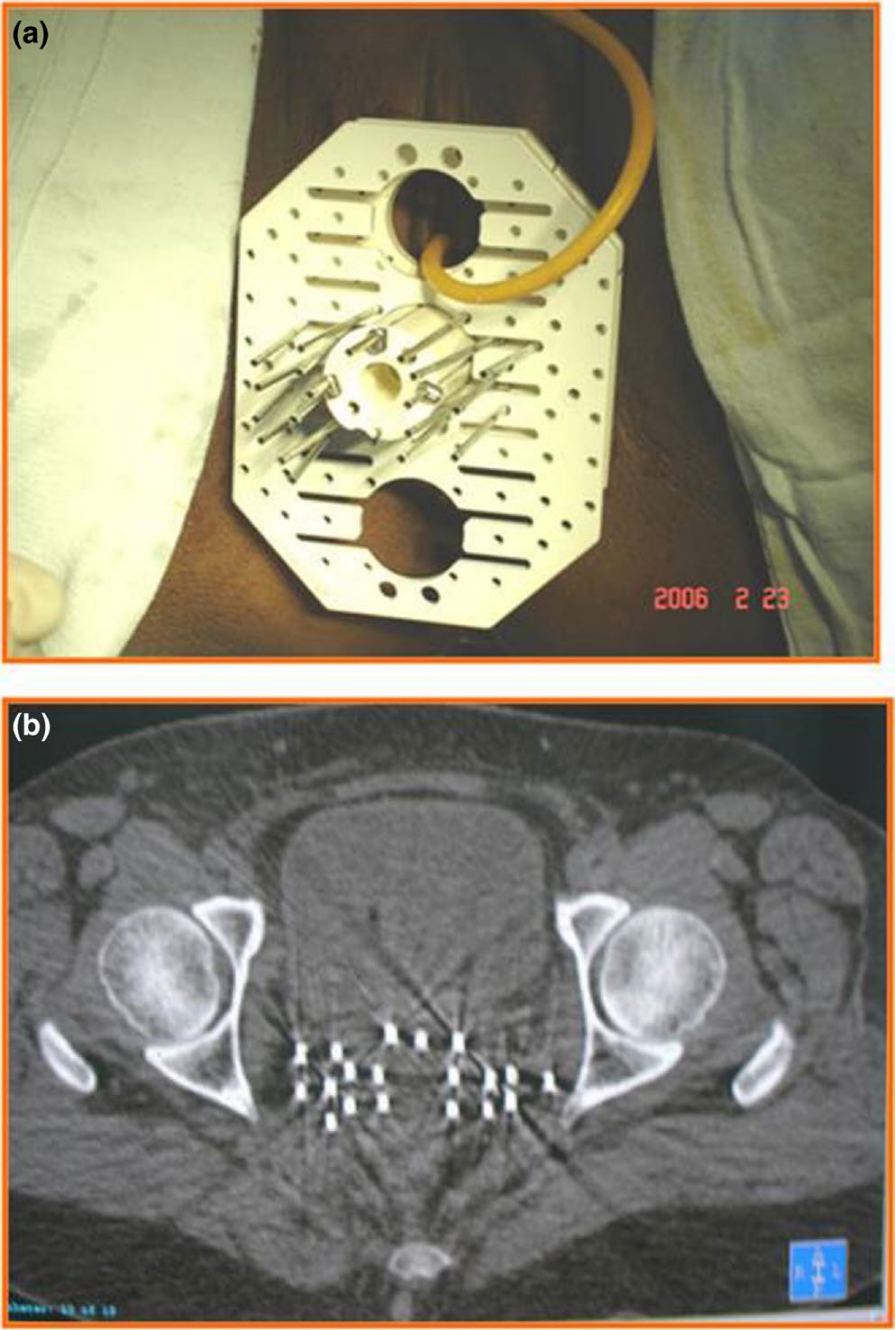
Interstitial brachytherapy implant: (a) clinical image of a patient showing the perineal template and the steel needles; and (b) computed tomography image showing the brachytherapy needles inserted into the pelvis.
